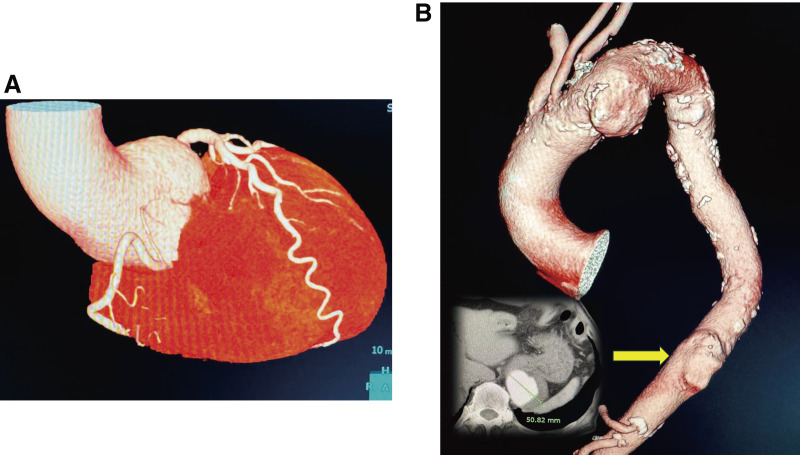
Preoperative imaging. (A) A contrast-enhanced CT scan showing dilation of the sinus of Valsalva and ascending aorta, with a maximum diameter of 60 mm. (B) A contrast-enhanced CT scan demonstrating a saccular aneurysm in the lower part of the aortic arch (arrow), measuring approximately 50 mm, with mild calcification and mural thrombus. CT: computed tomography
